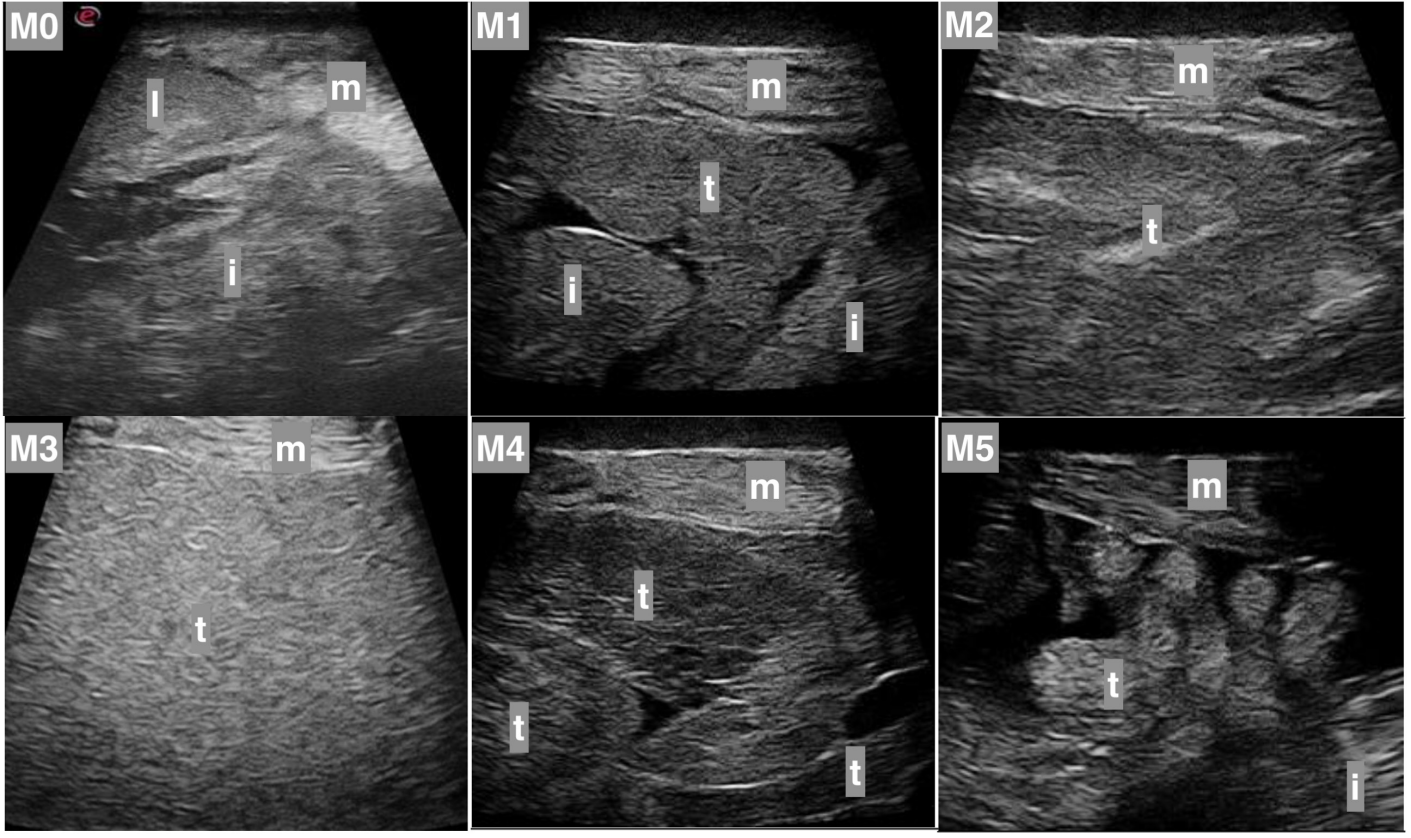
Ultrasound view of lumpfish testes. M0 to M5 are ultrasound gonadal stages from immature to spent testes. Apart from testes (t), liver (l), intestines (i), and muscles (m) are also shown. The ultrasound gonadal categories are described in Table 2.
